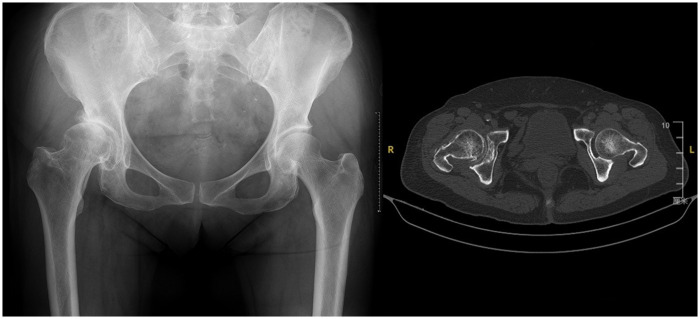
Anteroposterior x-ray showed the right femoral head has shifted outward and upward, with an irregular shape and irregular edges. There is also bone hyperplasia at the edge of the acetabulum.
